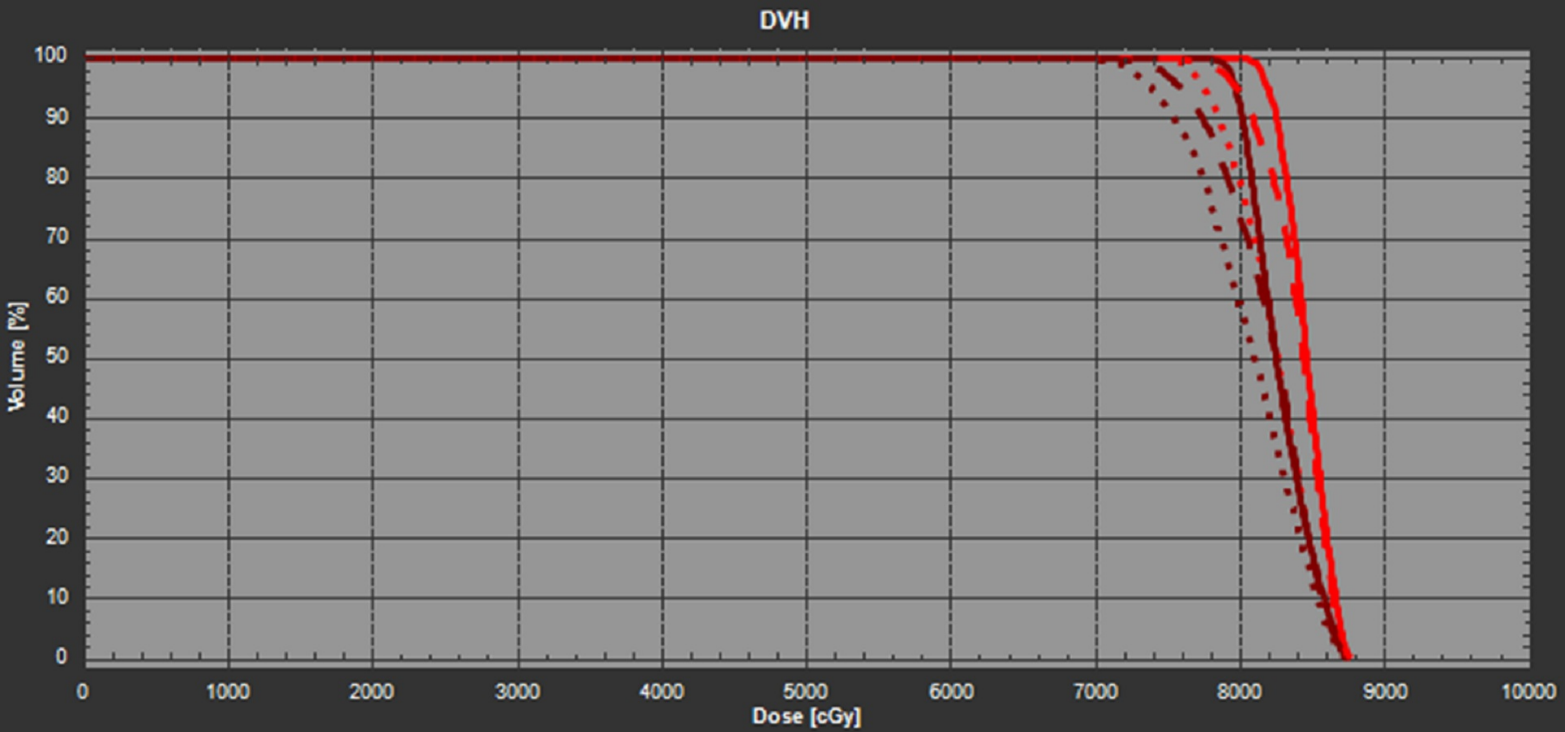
The DVH of the RR plan in the planning CT (solid line), VFCT1 (dotted line), and VFCT2 (dashed line). The red lines denote the iGTV and the dark brown lines denote the CTV. CT: Computed tomography; CTV: Clinical target volume; DVH: Dose volume histogram; iGTV: internal gross target volume; RR: Roll robust; VFCT: Verification CT.
